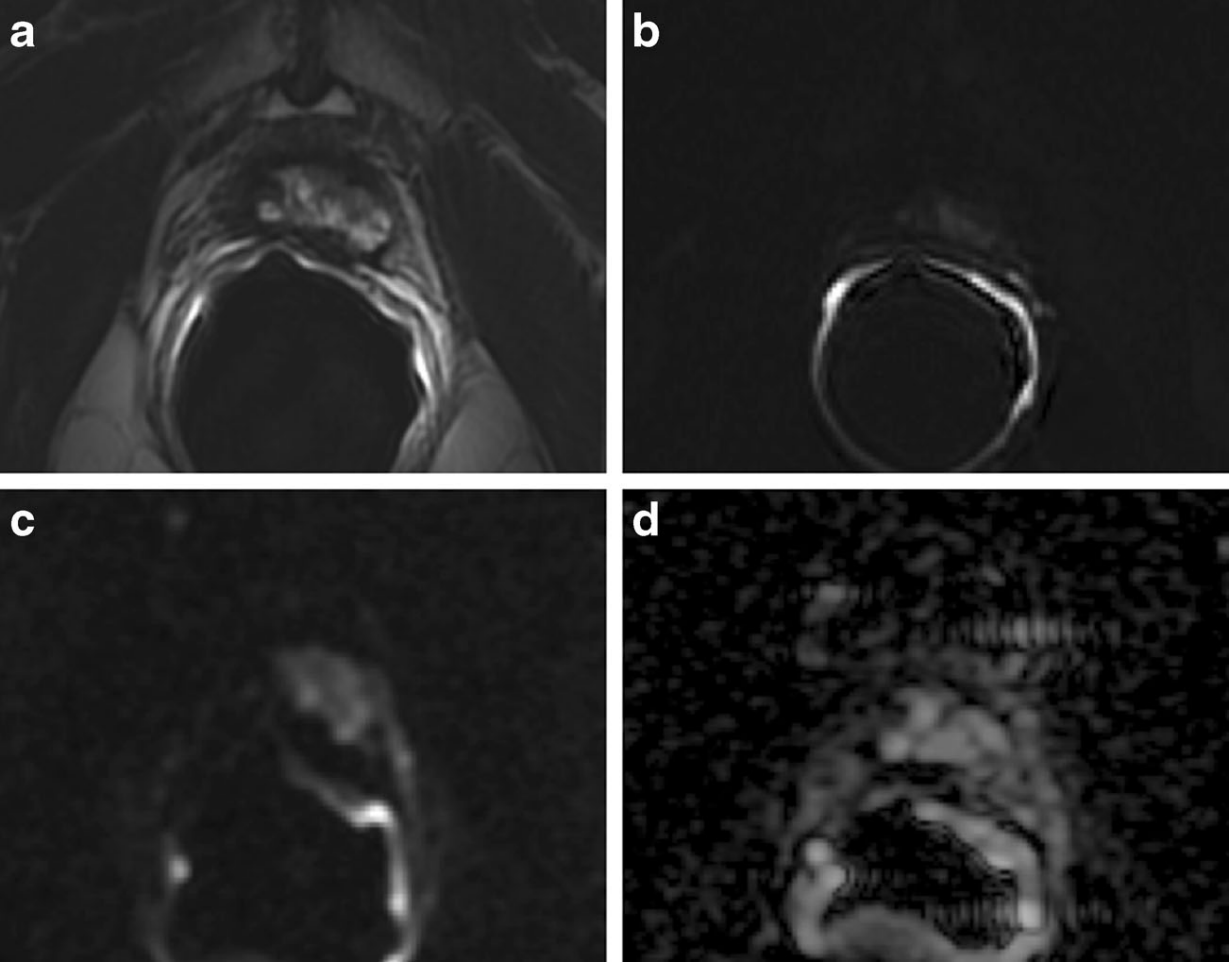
Post-HIFU treatment. a Axial T2-weighted fast spin-echo image showing an area of intermediate signal intensity in the anterior left portion of the gland after 3 months post-HIFU treatment, suspected for recurrence. b Axial perfusion gradient-echo T1-weighted image shows no significant enhancement in the suspected area. c Axial DWI image with b value 1000 mm^2^/sec, showing a higher hyperintense area in the anterior left portion of the prostate, d ADC map image showing no areas of significant restriction of water diffusivity, corresponding with post-HIFU treatment outcomes
